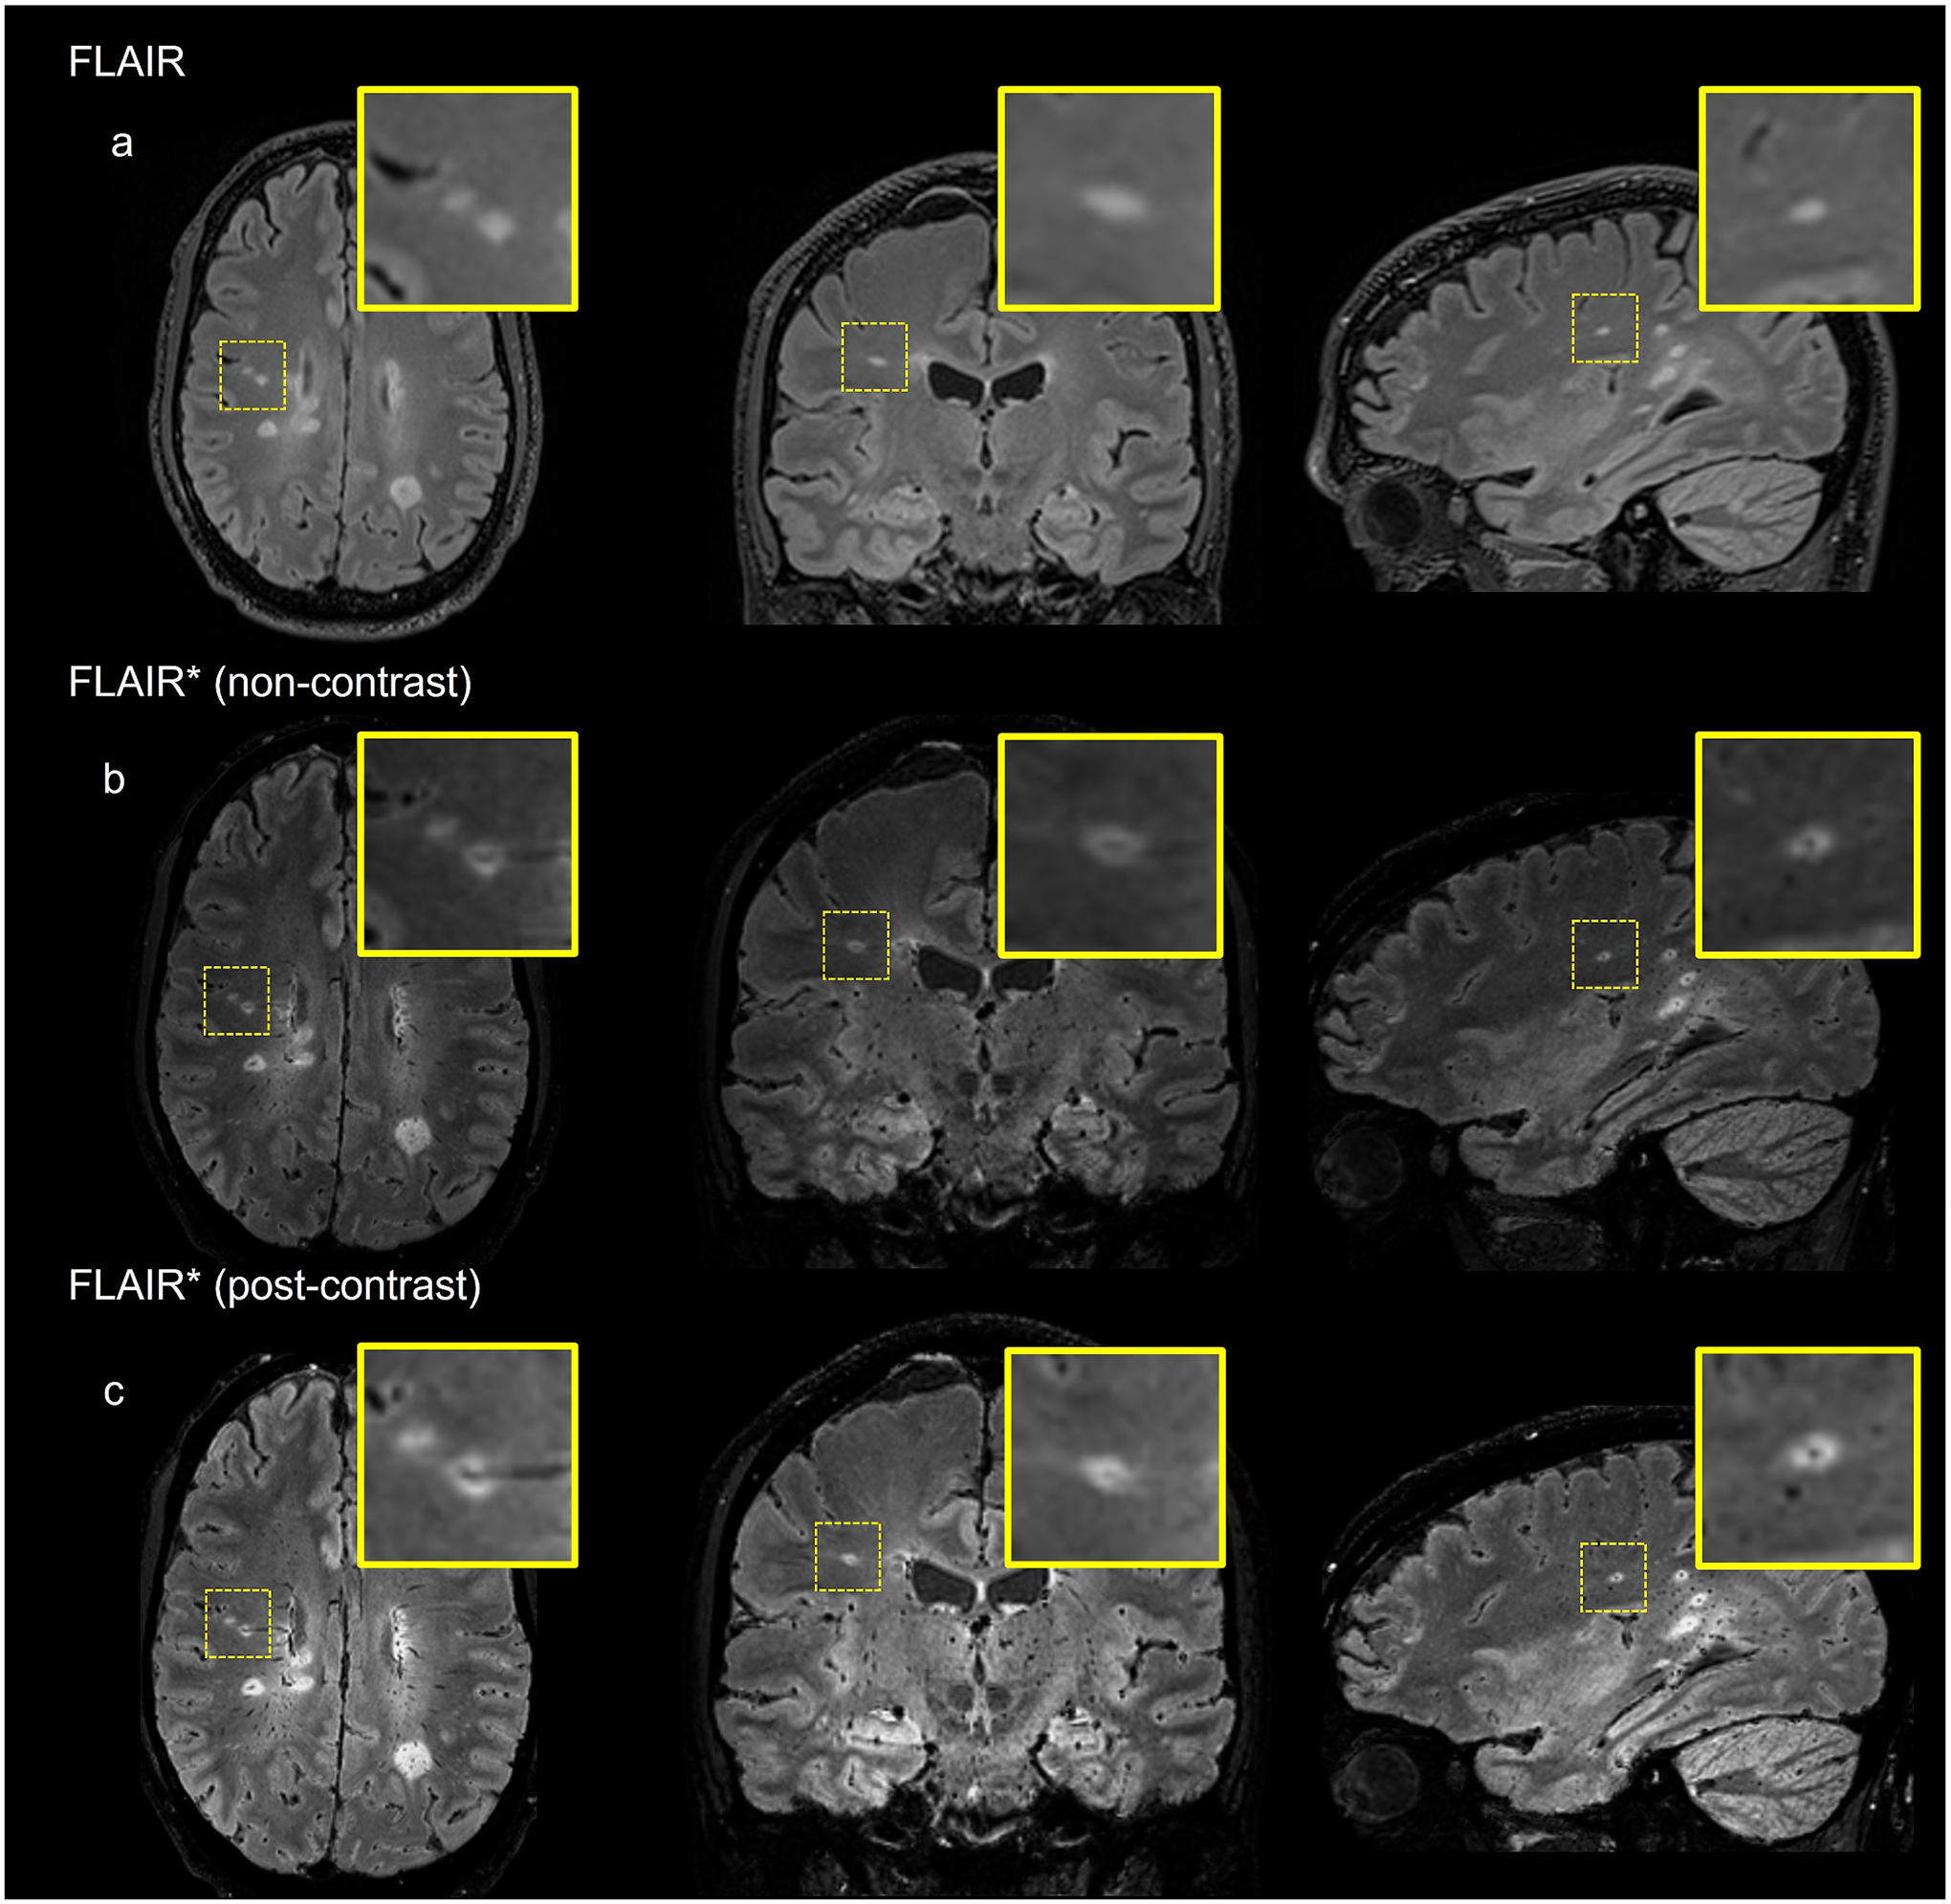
Example of a FLAIR hyperintense lesion without a central hypointensity (a), but a clear CVS on non-contrast (b) and post-contrast FLAIR* (c).
